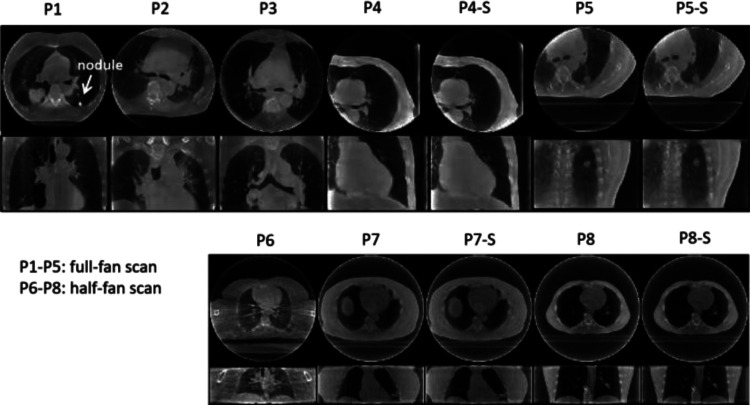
Reference-frame CBCTs reconstructed by PMF-STINR for the patient study. The upper and lower rows show the reconstructions from full- and half-fan scans, respectively.
